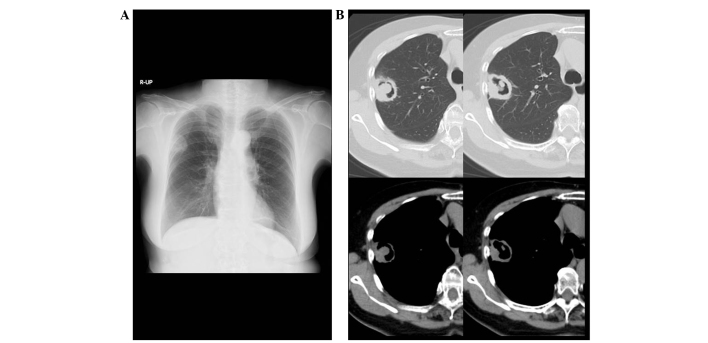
Initial chest X-ray and CT findings. (A) Plain chest X-ray showing a nodule 3.5 cm in diameter next to the pleura in the right upper lung. (B) Chest CT revealing a lung cavity with ball-like structures and air crescent signs. CT, computed tomography.
